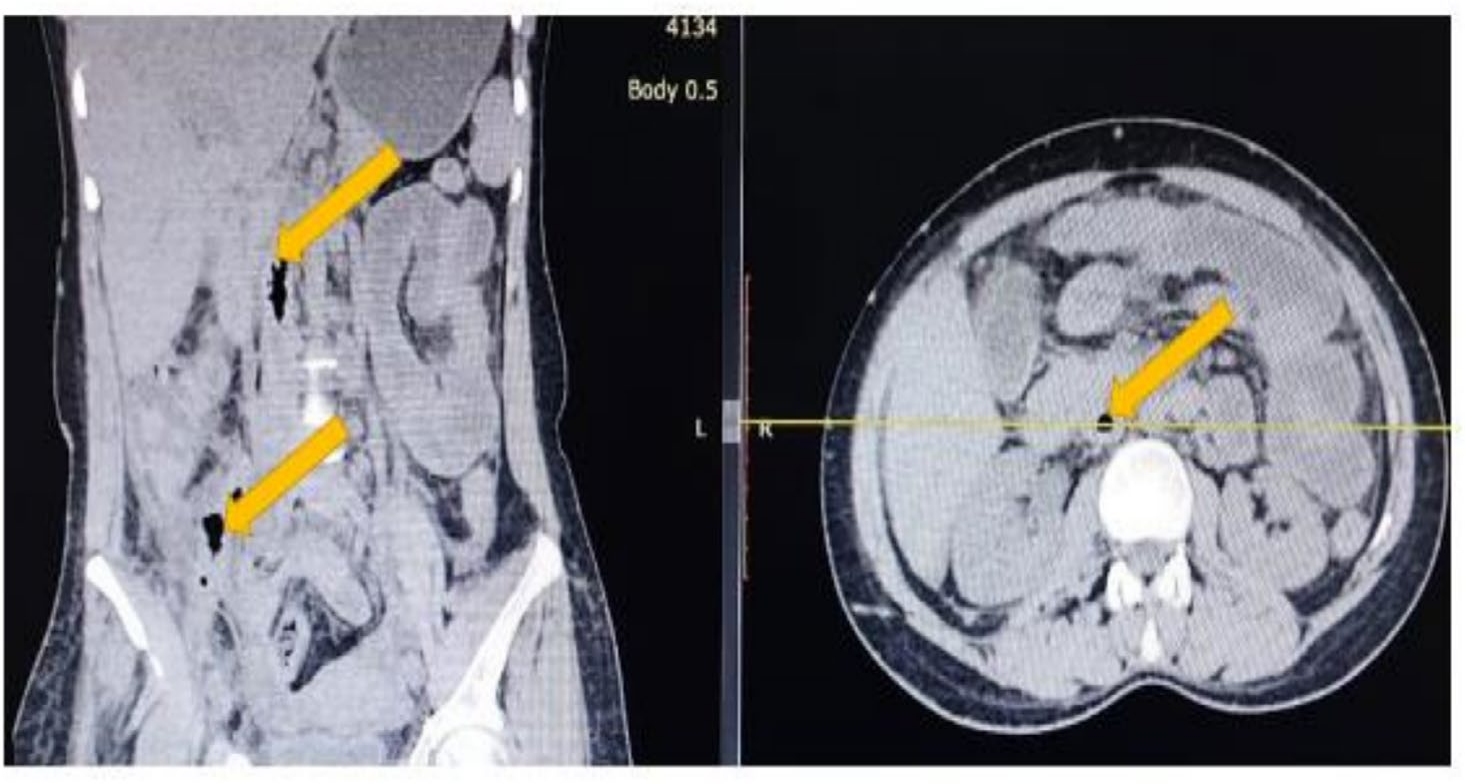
Bilateral psoas abscesses. A non‐contrast axial computed tomography (CT) image demonstrating large, complex collections within both psoas muscles, consistent with abscesses. The right‐sided collection is significantly larger and contains a substantial volume of gas.
